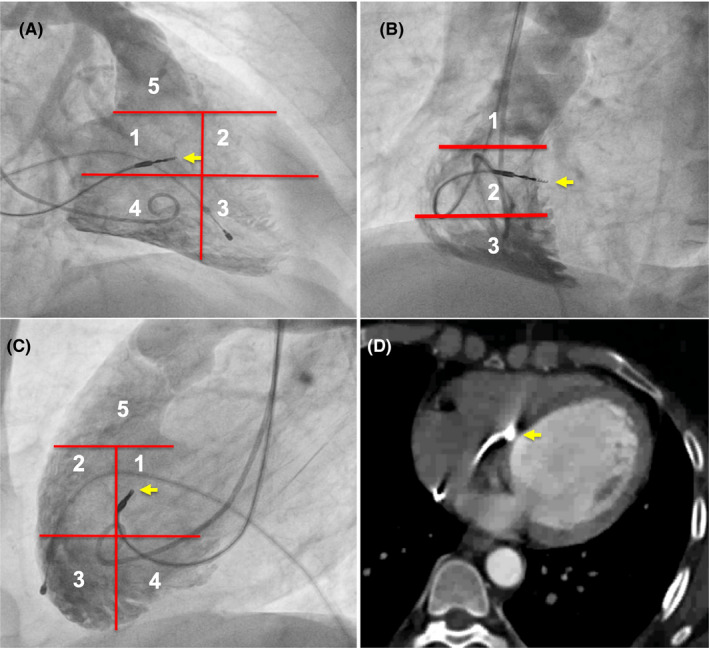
Right ventricular angiographic and CT scan of the mid‐septal lead position. RV angiography (A‐C) along with the described segments and the comparative CT scan (D) in diastole. Panel demonstrates (A) RAO 30° with 5 segments, (B) LAO 40° with 3 segments, (C) left lateral with five segments, and (D) CT scan. Note the tip of the ventricular lead (yellow arrow) in segment 1 in RAO 30° and LL views and the middle segment in the LAO 40° view in panels (A‐C), and the lead tip in the mid‐septal region on the CT scan. LAO, left anterior oblique view; LL, left lateral 90° view; RAO, right anterior oblique view; RV, right ventricle
